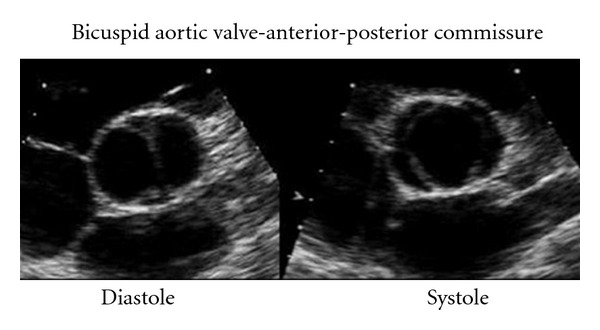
The bicuspid valve in the parasternal short axis view.
